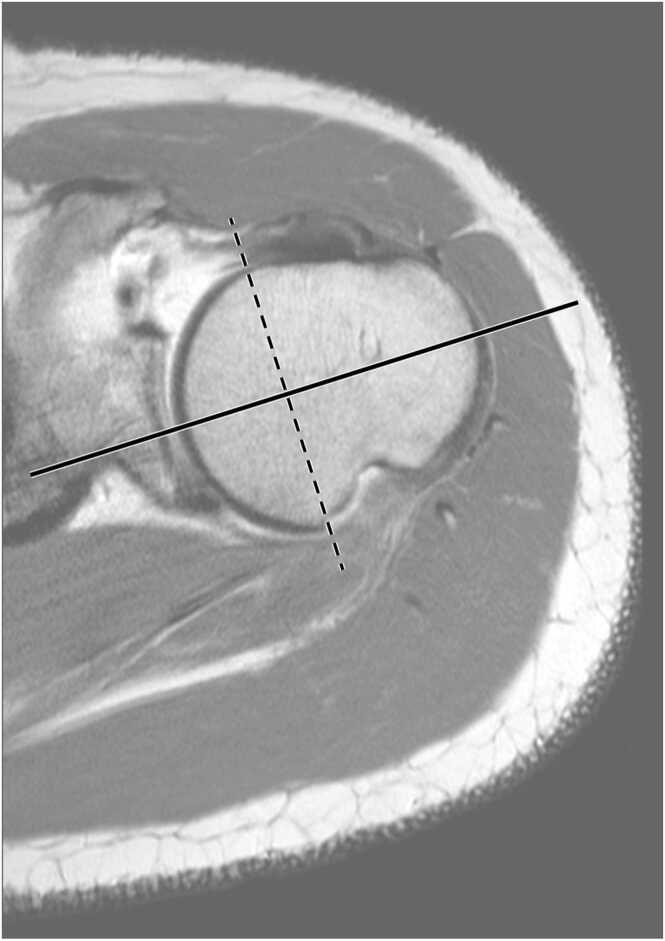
Determination of the humeral head axis on MRI sections by Bernageau and Godefroy’s method [12], [42] in a case with a Hill-Sachs lesion.
